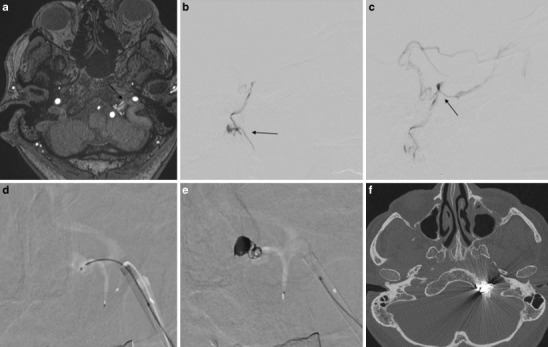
Case #4: a MR angiography source image from three-dimensional time-of-flight shows abnormal vascularization in the left hypoglossal canal compatible with a DAVF of the anterior condylar vein (*arrow*). b Lateral view after injection of the right ascending pharyngeal artery shows a DAVF of the anterior condylar vein with a venous pouch (*arrow*) located medial to the jugular bulb. c Lateral view after injection of the right ascending pharyngeal artery shows a DAVF of the anterior condylar vein with a venous pouch at the point of the fistula (*arrow*) and also reflux to a petrosal and sphenopalatinal cortical vein. d AP view shows the microcatheter in the venous pouch of the DAVF of the anterior condylar vein through a transvenous approach via the left jugular vein. e AP view after injection of the left ascending pharyngeal artery after coil occlusion of the left anterior condylar vein shows the complete occlusion of the DAVF. f Axial CT scan of the skull base confirmed the location of the coils in the hypoglossal canal
